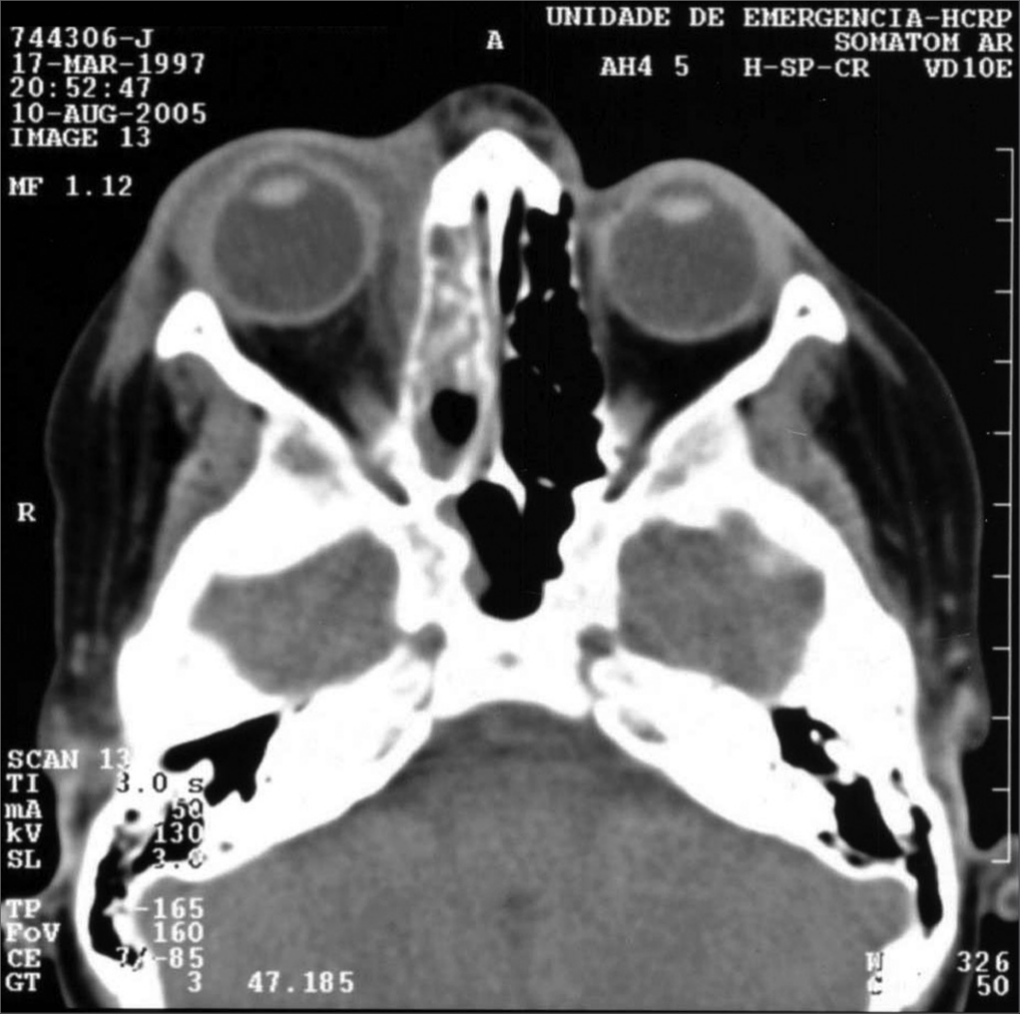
Axial view CT scan of the orbit showing orbitary cellulitis on the right side.
